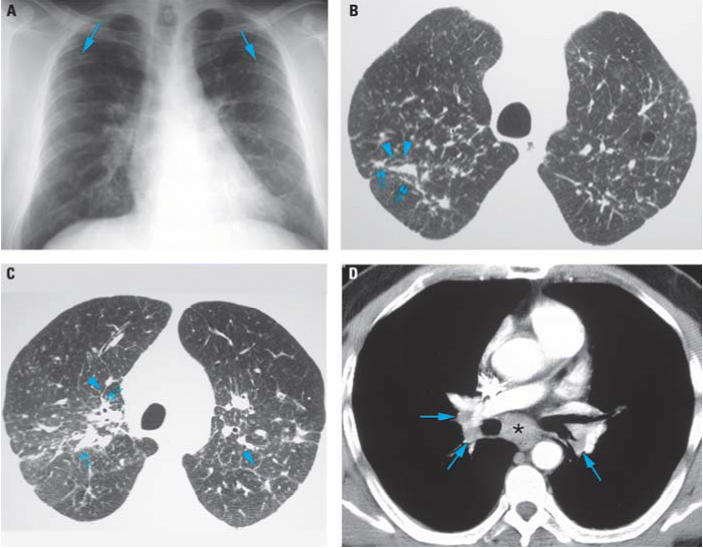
Chest radiograph (*A*) and HRCT images (*B–D*) of patient’s lungs. (*A*) Frontal chest radiograph showing mild symmetric linear and reticular opacities (arrows) in the upper lobes bilaterally; these opacities are associated with bilateral hilar prominence, suggesting lymphadenopathy. Note the upper lobe distribution of the findings as well as the absence of associated pleural thickening. (*B*) Axial HRCT image through the lung apices shows bilateral, patchy bronchovascular thickening with a nodular appearance (small double arrows). Nodular interlobular septal thickening is also present (arrowheads). (*C*) Axial HRCT image through the upper lungs slightly caudal to (*B*) shows bilateral, patchy bronchovascular thickening with a nodular appearance (small double arrows). Mild interlobular septal thickening is again present (arrowheads). Note the posterior distribution of abnormalities. *D*) Contrast-enhanced axial CT image shows subcarinal (*) and mild bilateral hilar (arrows) lymphadenopathy.
